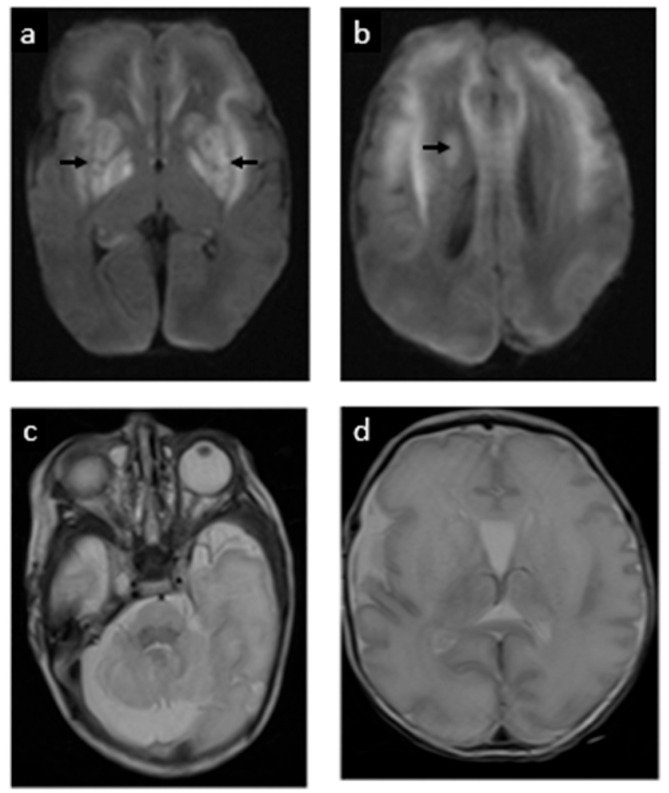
Postnatal MRI at 33 weeks 3 days gestational age equivalent (day 5 postnatally): postnatal MRI revealed acute injury to the basal ganglia, shown as extensive restricted diffusion/cytotoxic edema (white areas in images (a,b), signaled with arrows). (c) The cerebellum has a normal appearance. (d) Simplification of supratentorial gyral pattern, a reflection of prematurity.
